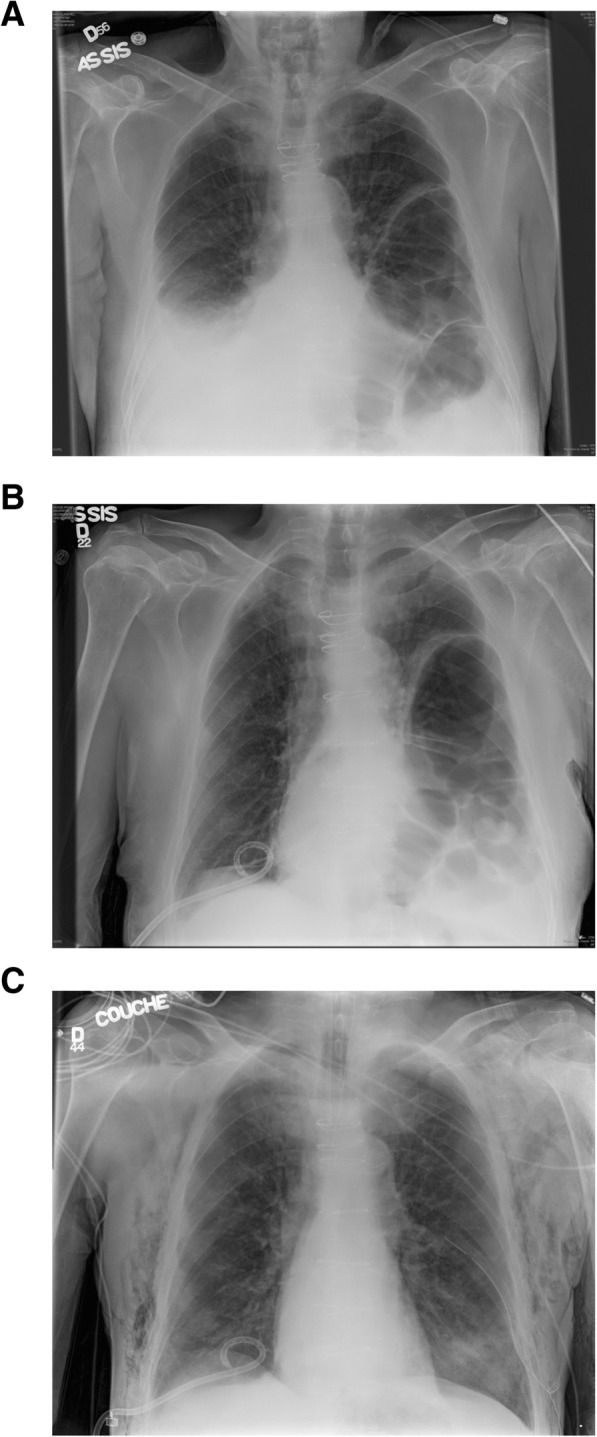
Anterior to posterior chest roentgenogram showing: (a) recurrent right chylothorax combined with the left intrathoracic herniated colon (upright sitting position); (b) right pigtail catheter insertion and chylothorax drainage (upright sitting position); (c) resolution of both the chylothorax and the herniated colon (supine position)
